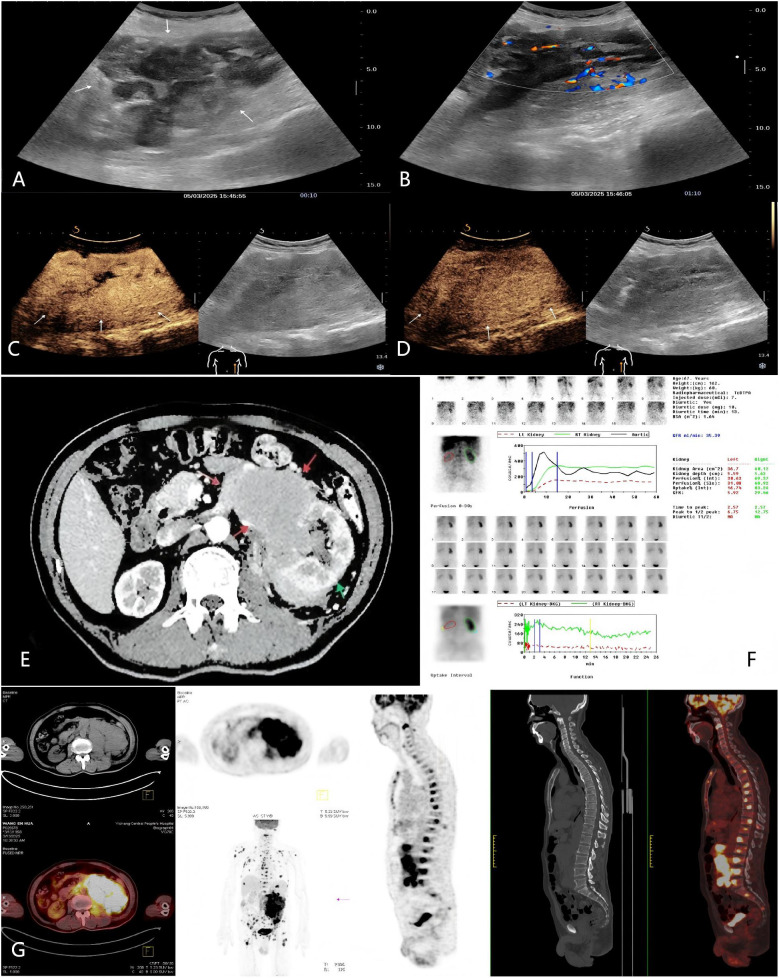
Multimodal imaging of renal extramedullary disease. (A) Conventional US showed a heterogeneous hypoechoic mass adjacent to the left kidney without calcification, with irregular shape and unclear boundaries (white arrow indicates the mass boundary); (B) Color doppler US showed abundant blood flow signals in the mass; (C) CEUS showed the mass exhibited high arterial-phase enhancement: heterogeneous enhancement in early phase. A small area of perfusion defect can be observed in the center of the mass; (D) In the late phase, contrast washout initiated earlier within the lesion relative to the renal parenchyma, whereas full clearance of microbubbles was delayed, leading to heterogeneous mild hypoenhancement of the mass; (E) CTU in the arterial phase showed that the left renal mass infiltrated the surrounding tissues with obvious exudation (red arrow indicates the mass, and green arrow indicates the left kidney); (F) ECT showed significantly reduced blood perfusion of the left kidney (red area), and normal blood perfusion of the right kidney(green area); (G)
